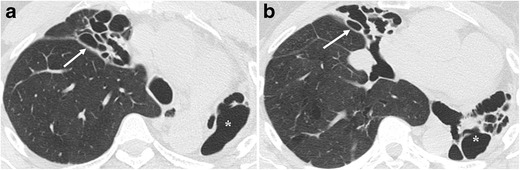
A 43-year-old female with TB history in her youth and recurrent infections presenting with fever. Sputum culture was postive for *Pseudomona aeruginosa*. (a) Non-enhanced CT shows total unilateral left lung destruction with bronchiectasias (asterisk) and lung atelectasis with saccular bronchiectasis in the right upper lobe (white arrow). (b) Right middle lobe atelectasis is also seen along with bronchiectasis (white arrow)
